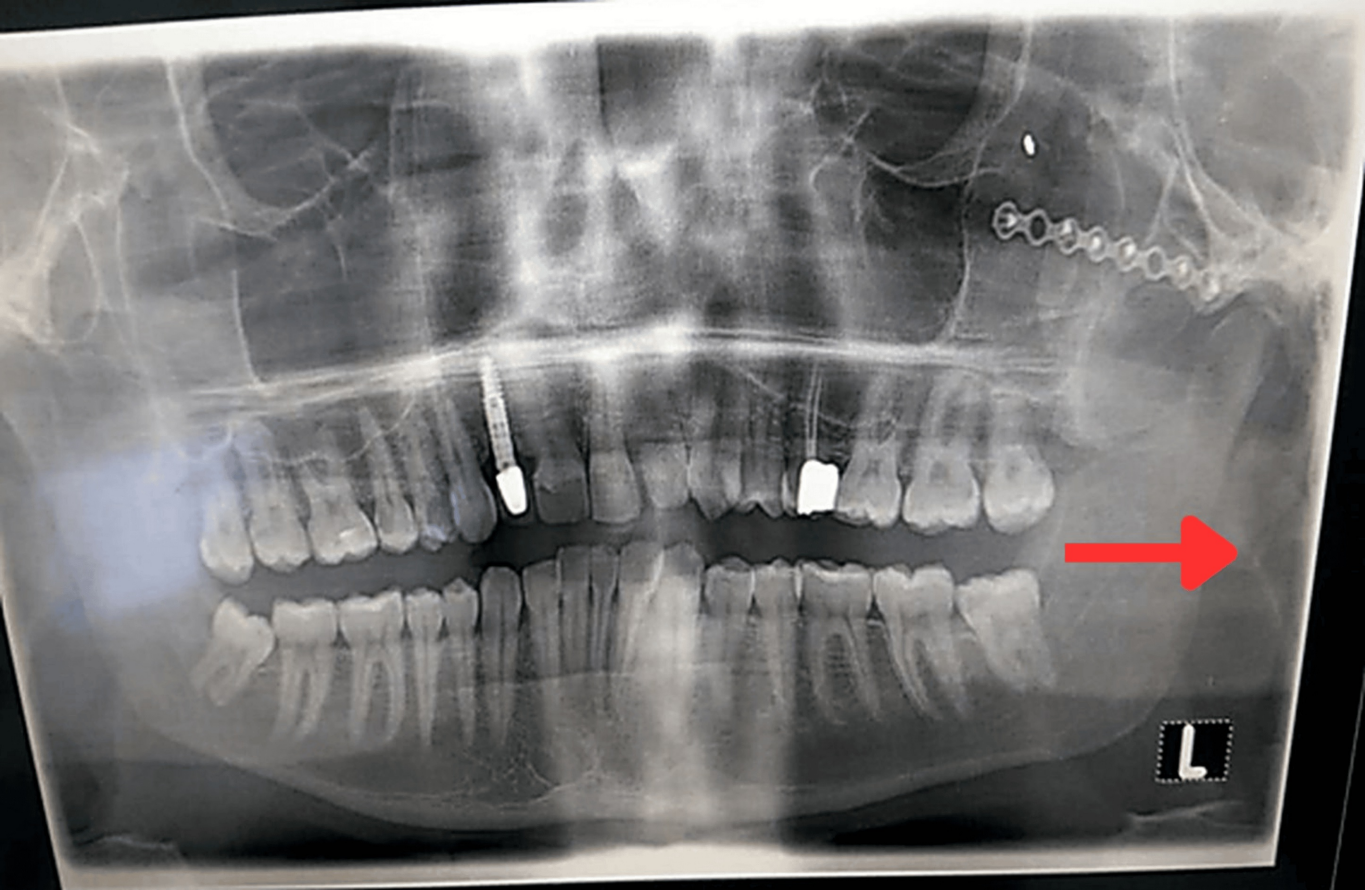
Panoramic radiograph at presentation demonstrating left zygomatic fixation hardware and heterotopic bone bridging the left mandibular ramus and zygomatic arch (red arrow), consistent with masseteric myositis ossificans.
